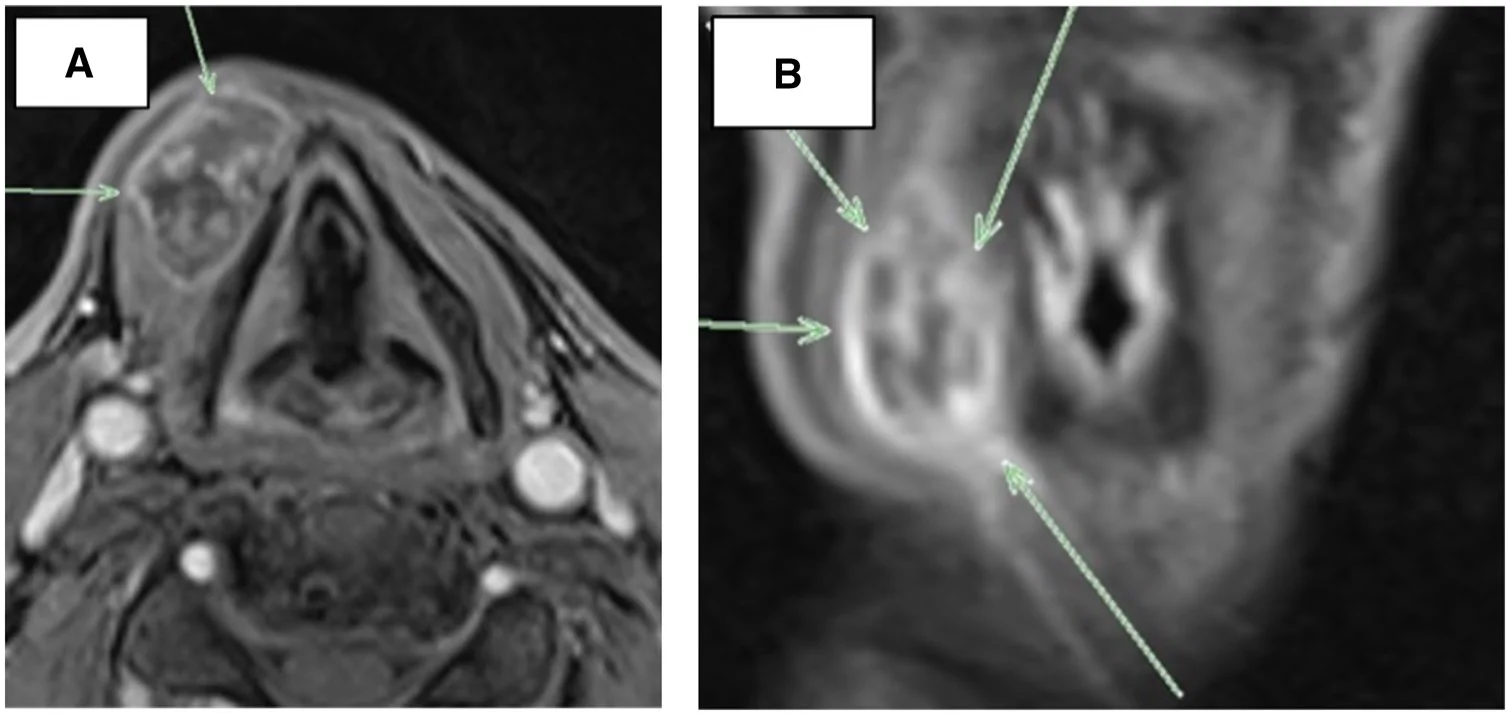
(A) Post-contrast axial T1-weighted MRI of the neck. Post-contrast T1-weighted imaging demonstrates heterogeneous enhancement of the mass lesion, which remains well defined and encapsulated without evidence of infiltration into adjacent soft tissues or surrounding structures. (B) Post-contrast coronal T1-weighted MRI of the neck. Coronal post-contrast imaging confirms the well-circumscribed enhancing lesion arising from the anterosuperior aspect of the right thyroid cartilage, with no invasion of the surrounding laryngeal structures.
